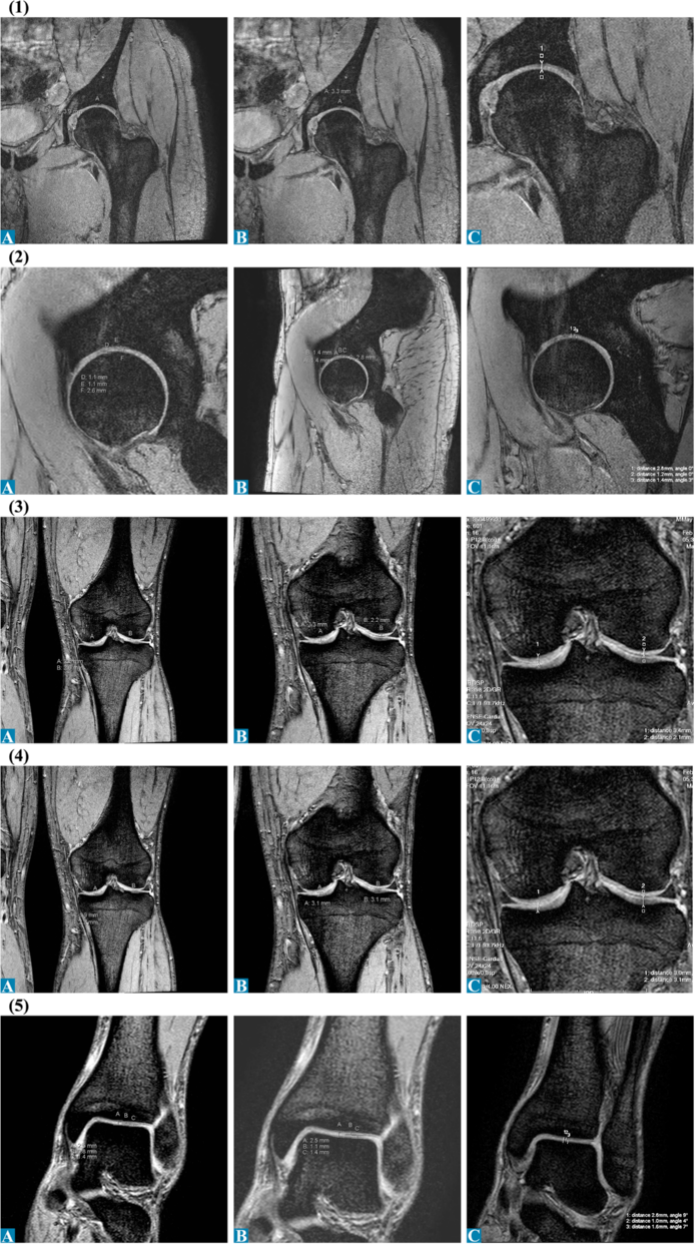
Articular cartilage thickness measurements of the left leg of radiologists A-C for 3.0 T MRI: (1) Hip coronal; (2) Hip sagittal; (3) Femur coronal; (4) Tibia coronal; (5) UAJ coronal.
